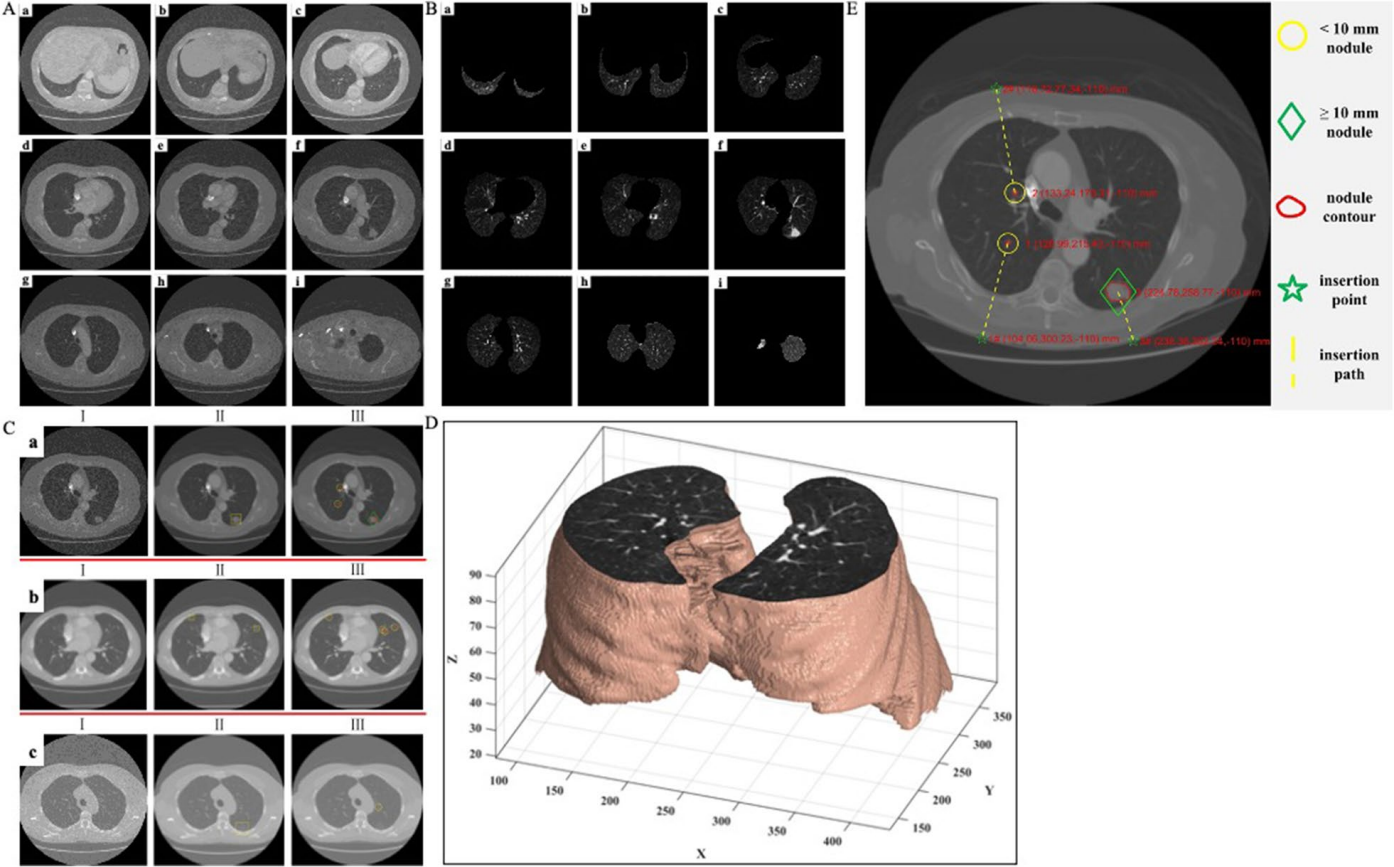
Pulmonary nodule detection. A CT gray images at different slices for the same patient. B Results of lung parenchyma segmentation corresponding to gray images in Fig. 4A. C Automatic recognition experiments for pulmonary nodules, where I to III represent original images, doctor-marked nodules, and diagnostic results in this paper, respectively. D A cross-section of the reconstructed 3-D model. E Path planning of nodules used for biopsy puncture.
